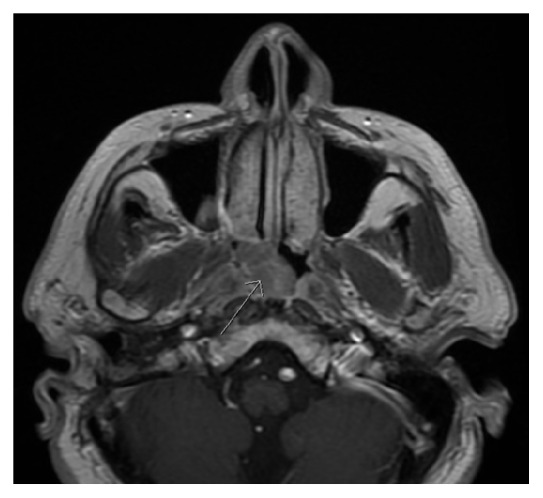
T1 weighted axial MRI demonstrating incidentally found polypoid, exophytic mass in the right nasopharynx.
